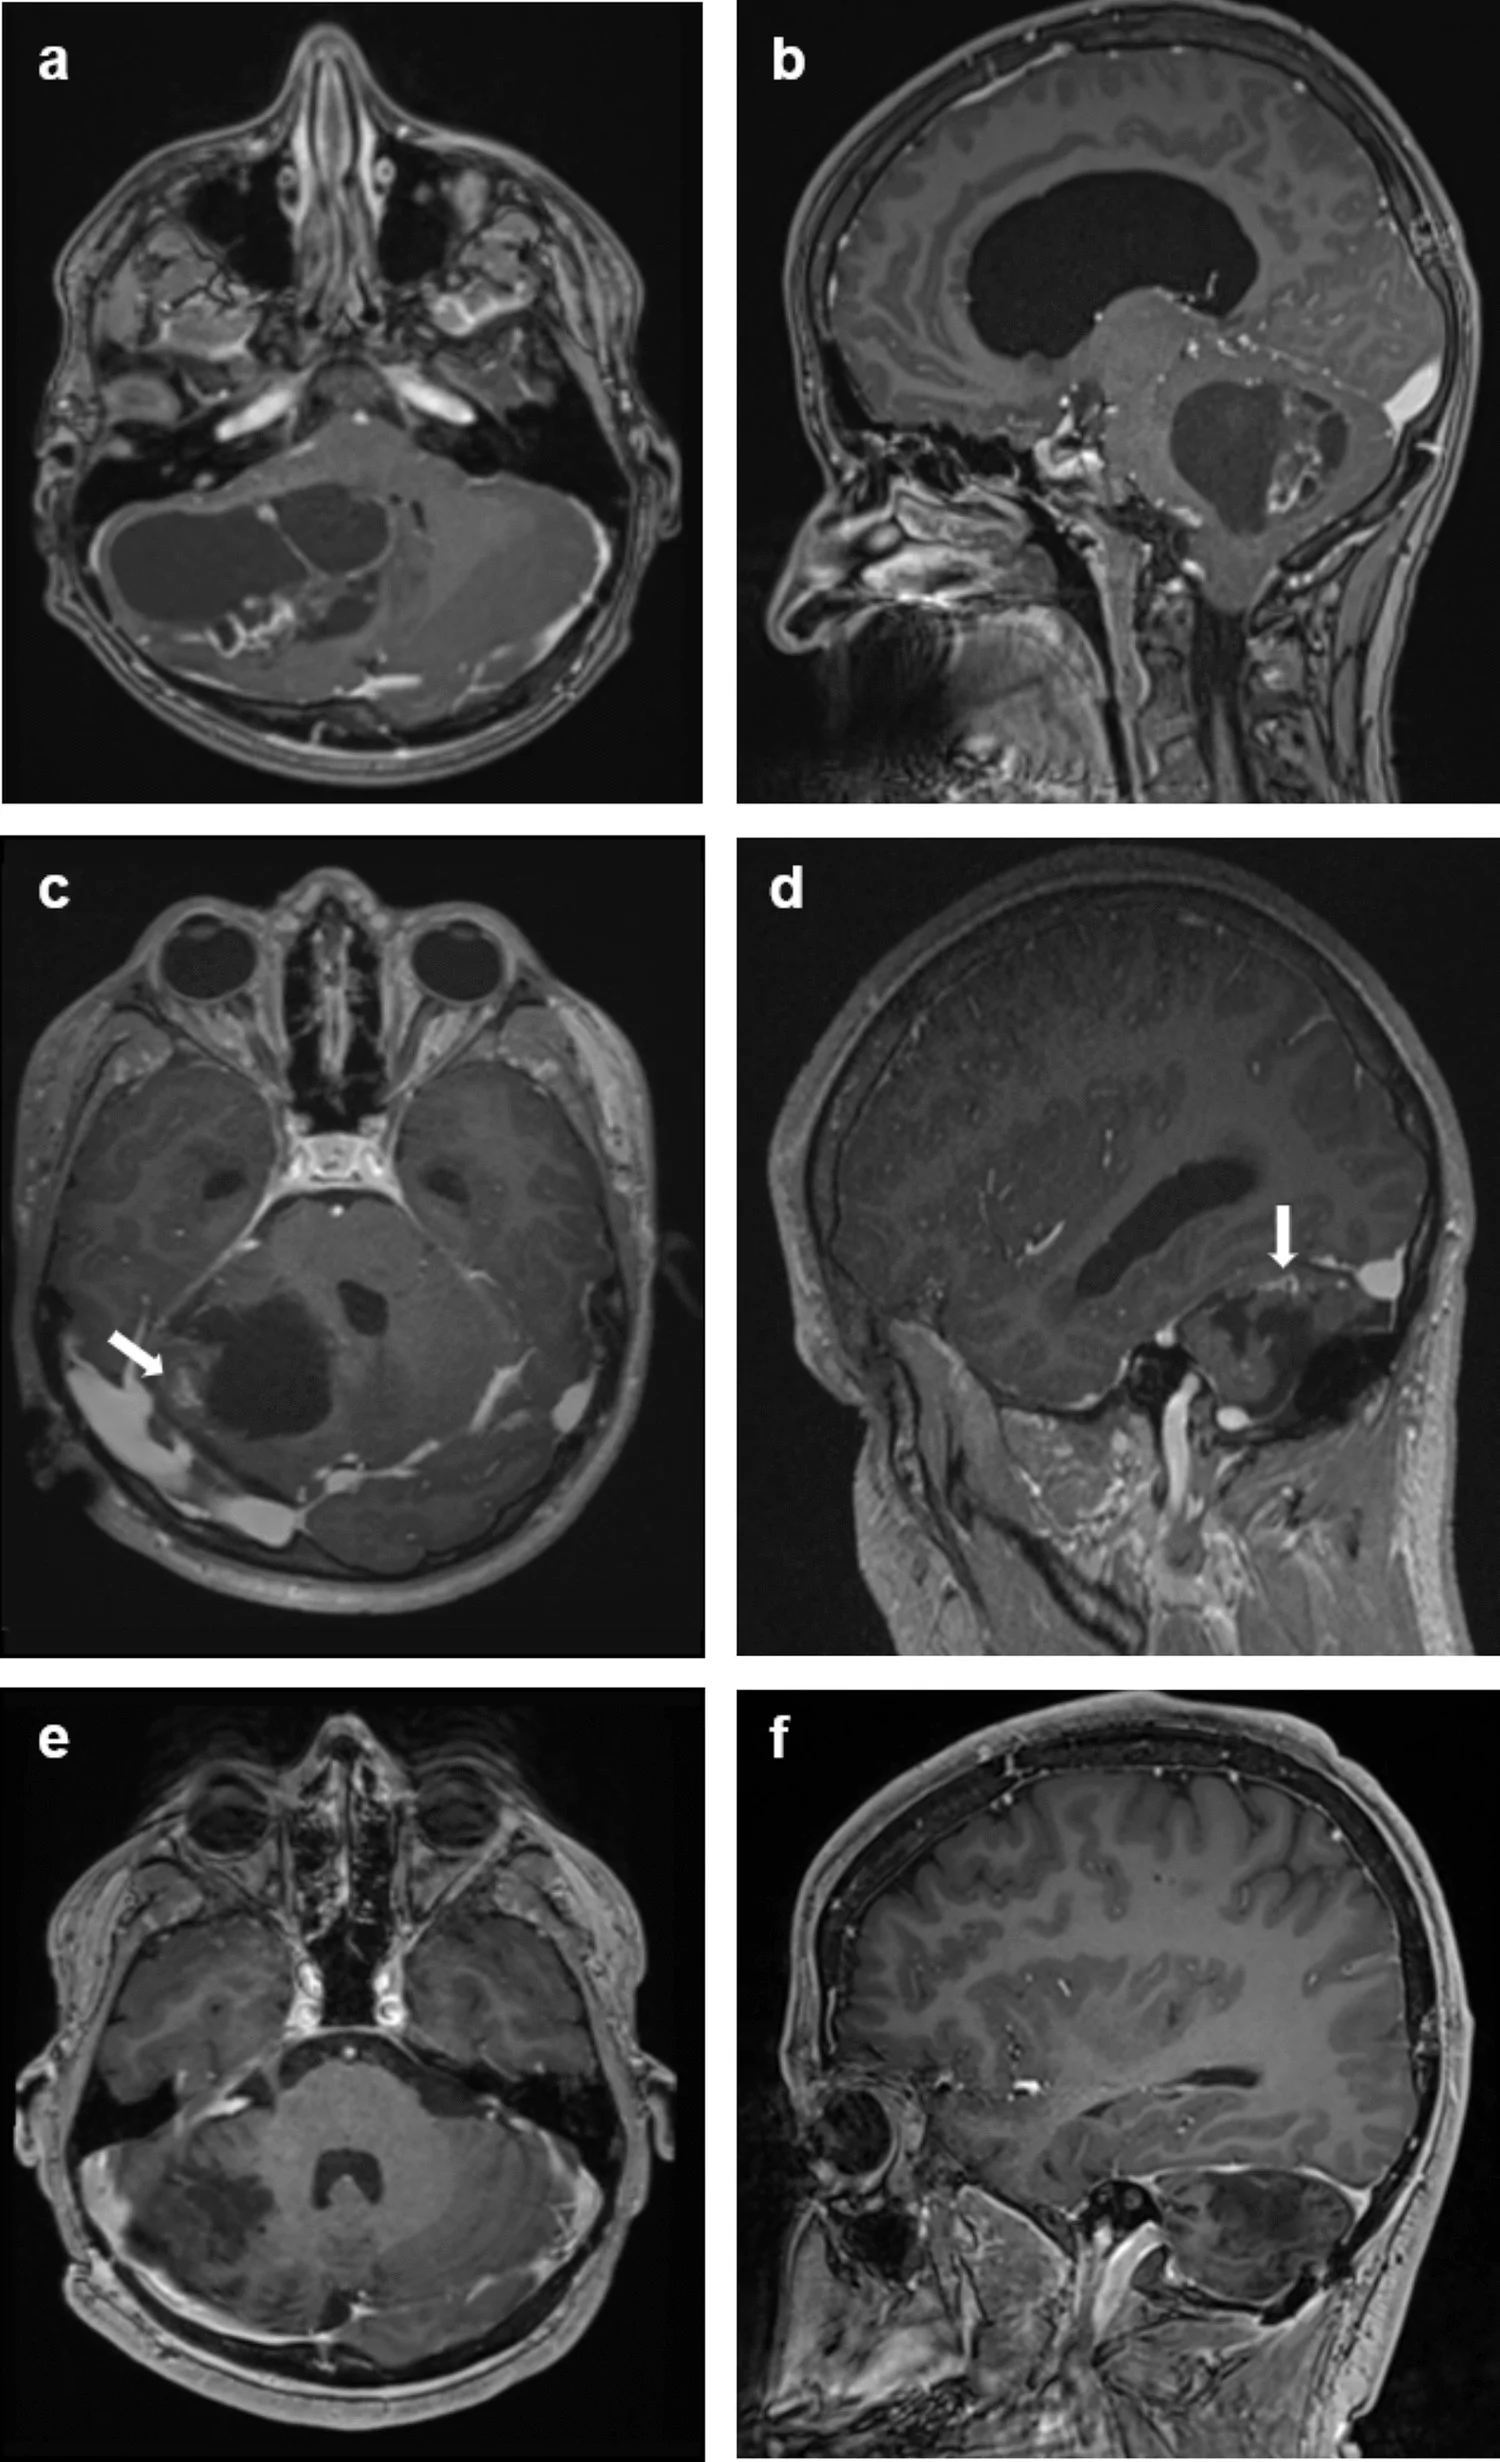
A 17-year-old patient presented with several months of headaches and vomiting occurring on an empty stomach. Preoperative MRI (T1-weighted axial [a] and sagittal [b]) demonstrated a cystic mass in the right cerebellum, suggestive of a pilocytic astrocytoma. Gross total resection (GTR) as the intended extent of resection (EoR). Intraoperative MRI (c, d) demonstrated residual contrast-enhancing tumor residues at the superolateral margin of the resection cavity (arrow), indicating that only a subtotal resection (STR) had been achieved. Additional tumor resection was therefore performed. Postoperative MRI (e, f), obtained the following day, confirmed complete tumor removal, resulting in a final EoR of GTR
